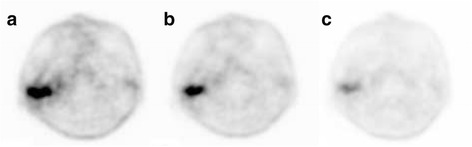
Representative ^18^F–BPA PET images in a 50-year-old man with squamous cell carcinoma of the external auditory canal. ^18^F–BPA PET images at (a) 30 min (SUVmax = 11.0, TNR = 5.0, TBR = 8.5), (b) 60 min (SUVmax = 8.9, TNR = 5.2, TBR = 6.9), and (c) 120 min (SUVmax = 6.3, TNR = 4.5, TBR = 5.3) after injection
